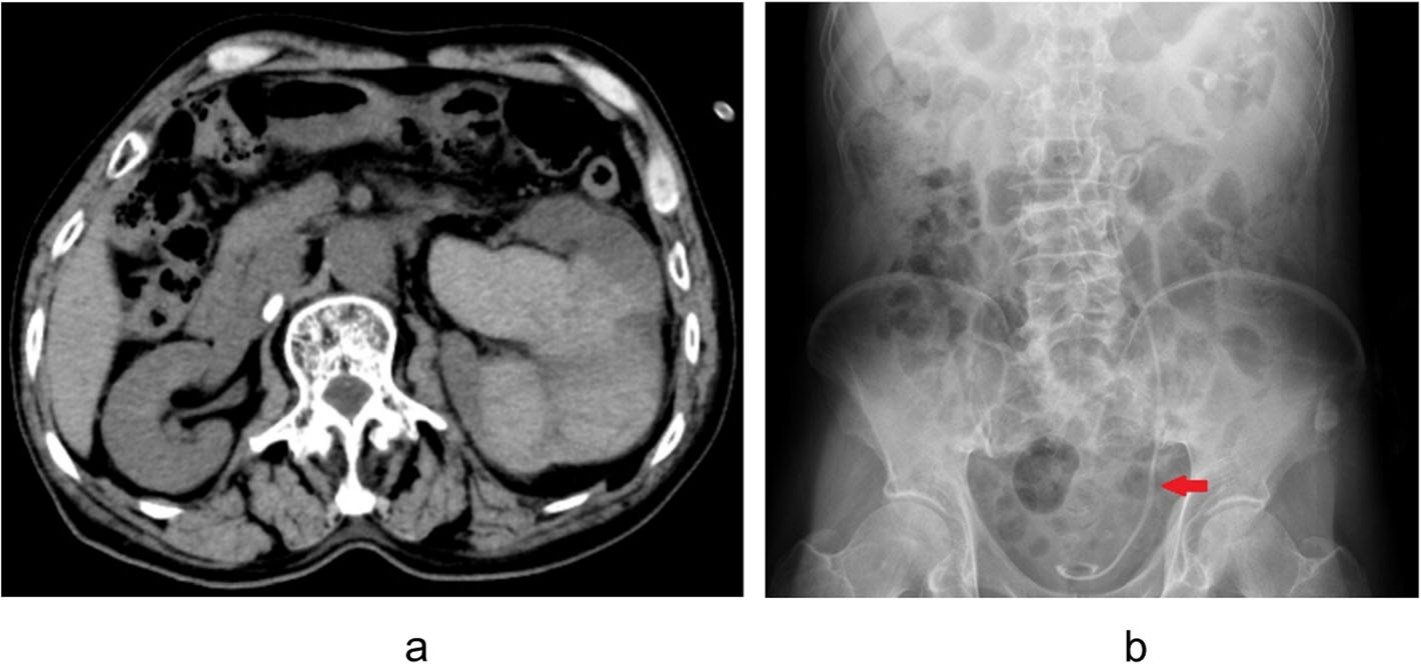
a CT revealed mild left hydronephrosis with blood clots. b KUB showed two ureteral stents in the left ureter
